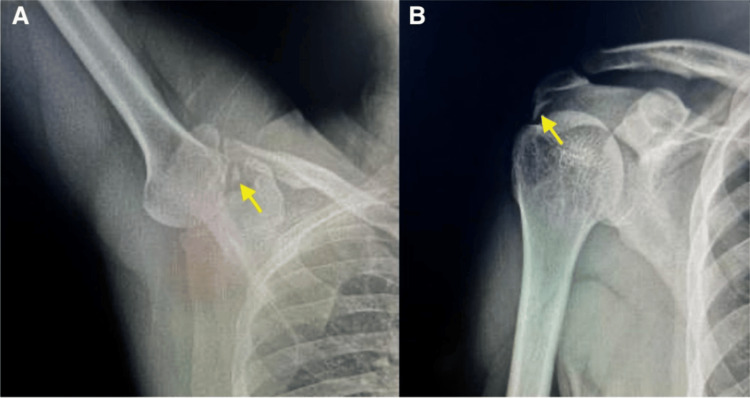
Right shoulder radiographs (A) axillary view showing calcific deposition within the anterior portion of the shoulder girdle (yellow arrow). (B) AP view showing the deposit in the subacromial space suggestive of calcific tendinitis within the supraspinous tendon (yellow arrow).
